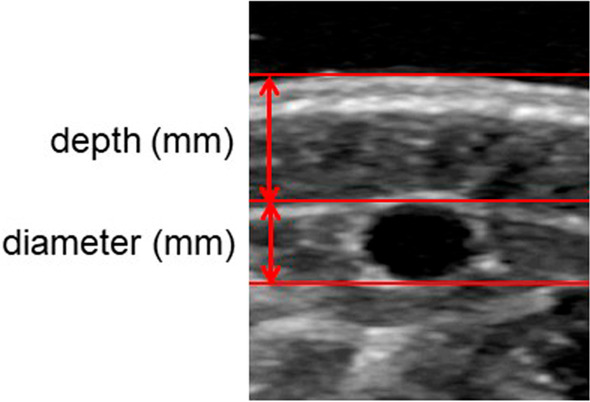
Ultrasound image of the superficial vein. Venous depth is the distance from the skin surface to the intima, and venous diameter is the distance between the intima
